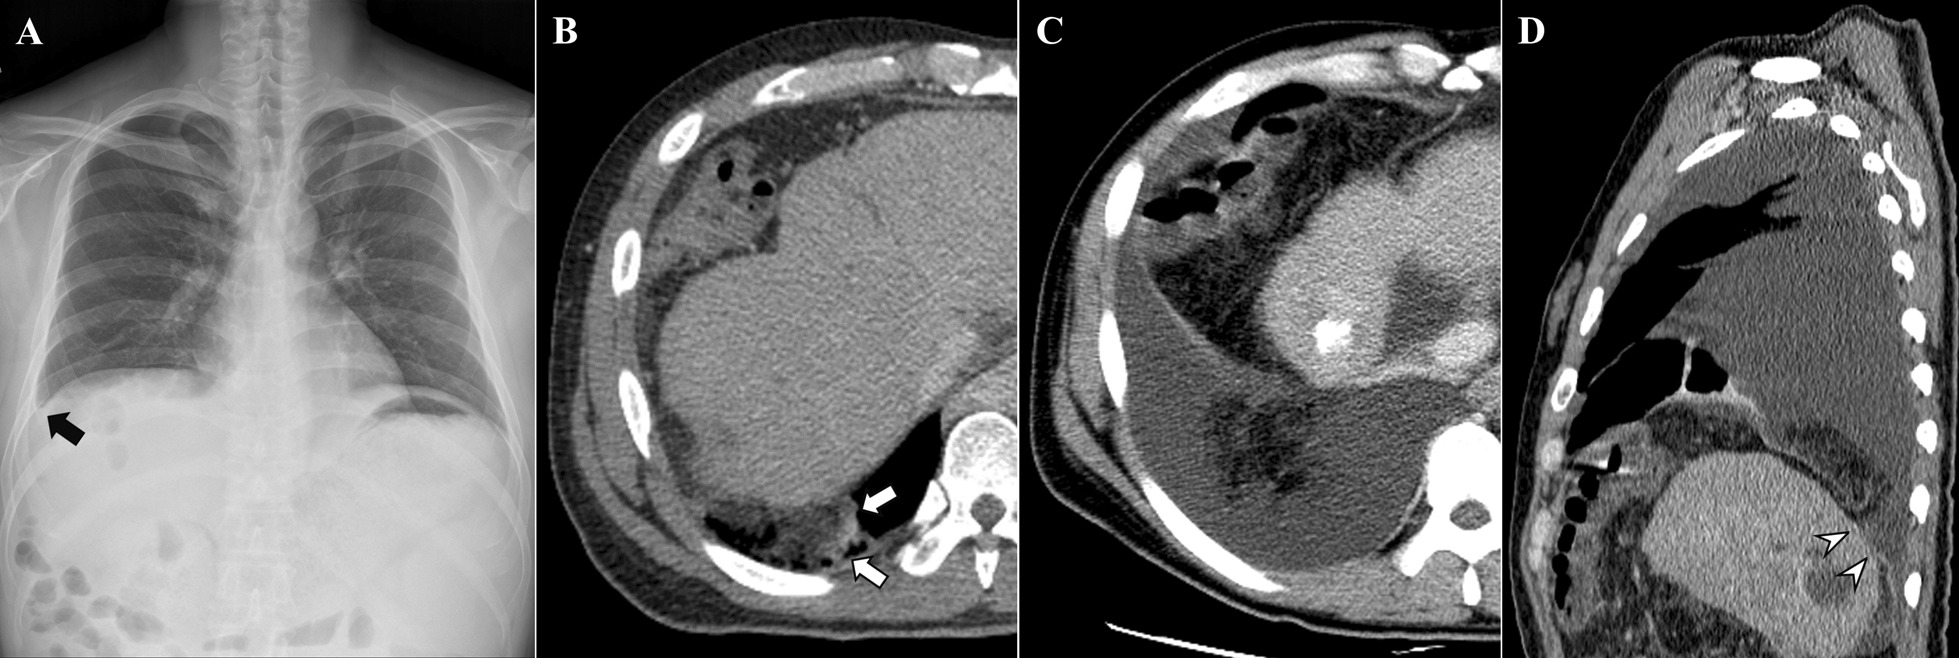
Diaphragmatic perforation and trans-diaphragmatic herniation. a Chest radiograph at admission shows mild blunting of the right costophrenic angle (black arrow). b Axial CT scan at admission reveals a mushroom-shaped diaphragmatic contour of the herniated omental fat (white arrows). c Follow-up axial CT scan after 2 months reveals a large pleural effusion and massive intrathoracic herniation of omental fat without organ entrapment. d Reformatted sagittal CT image shows direct discontinuity of the right hemidiaphragm (white arrowheads)
